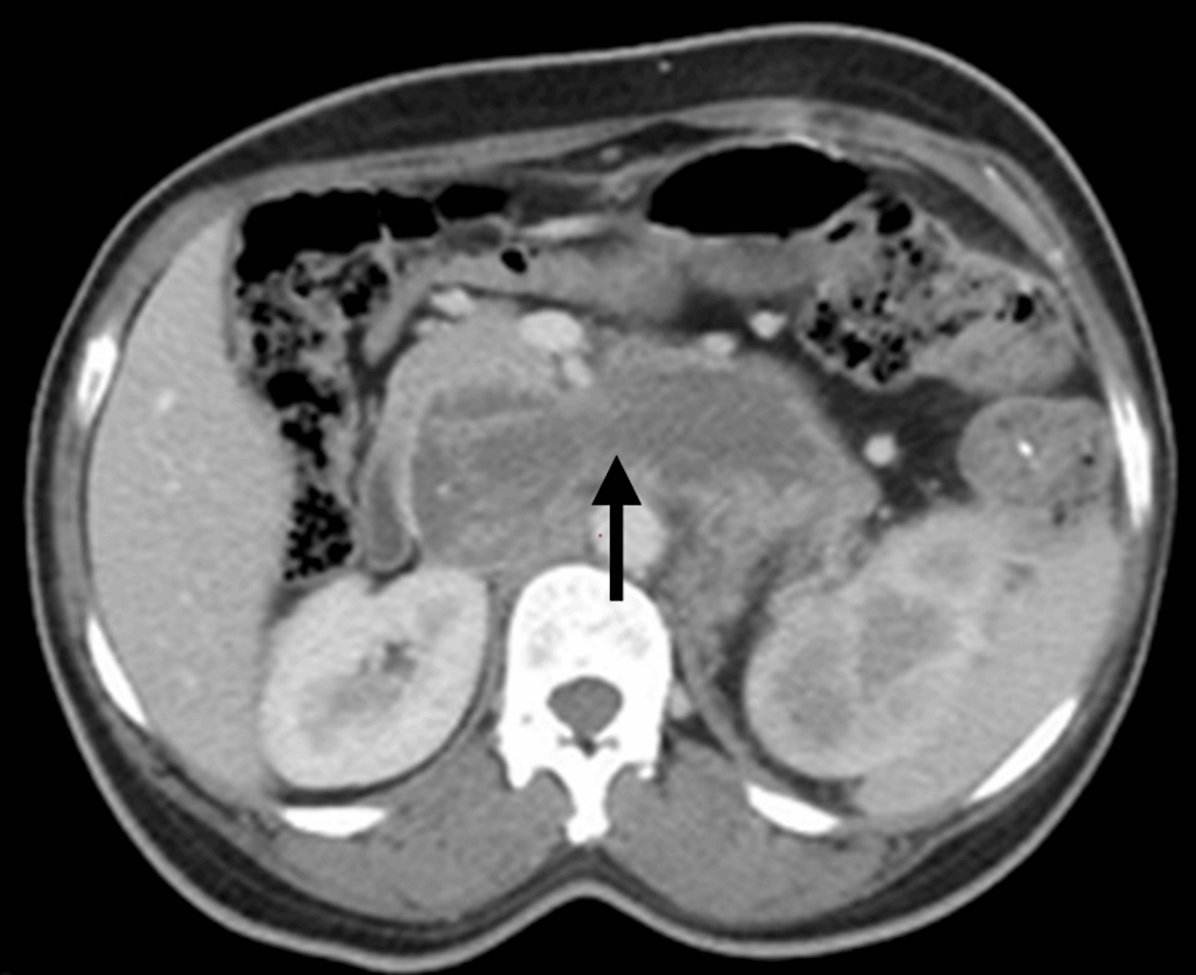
CECT abdomen axial section showing extension of tumor thrombus from the left renal vein into the IVC. CECT: contrast-enhanced computed tomography; IVC: inferior vena cava
